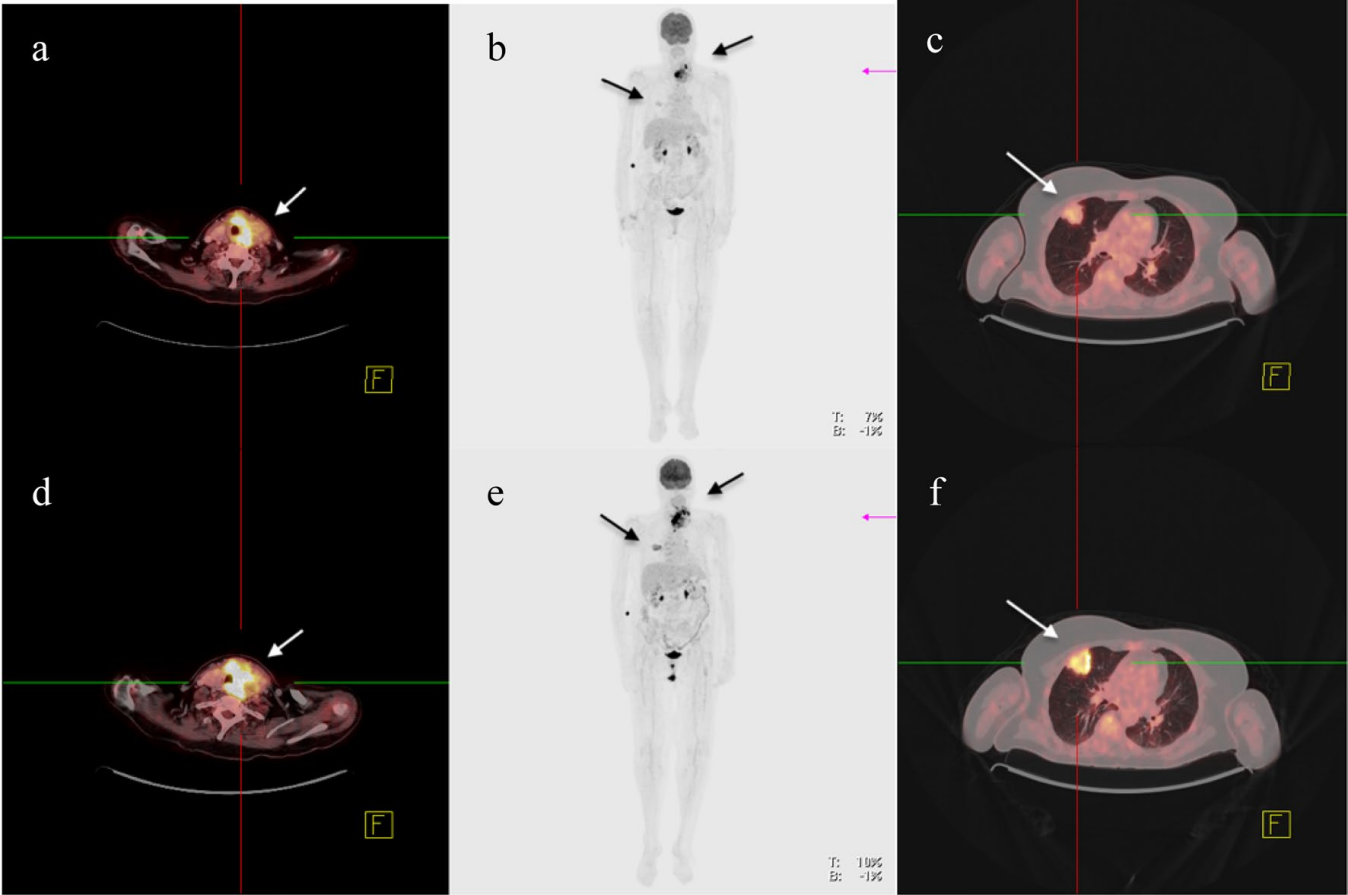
Patient 1# ATC of left thyroid lobe and isthmus and singular pulmonary metastasis of the middle lobe: ^18^F-FDG–PET/CT (d–f) at diagnosis and after neoadjuvant therapy (a–c). a/d Hypermetabolic mass affecting the left thyroid lobe and isthmus after neoadjuvant treatment/at diagnosis. b/e Primary tumor and pulmonary metastasis after neoadjuvant treatment/at diagnosis. c/f Pulmonary metastasis in the right middle lobe after neoadjuvant treatment/at diagnosis
